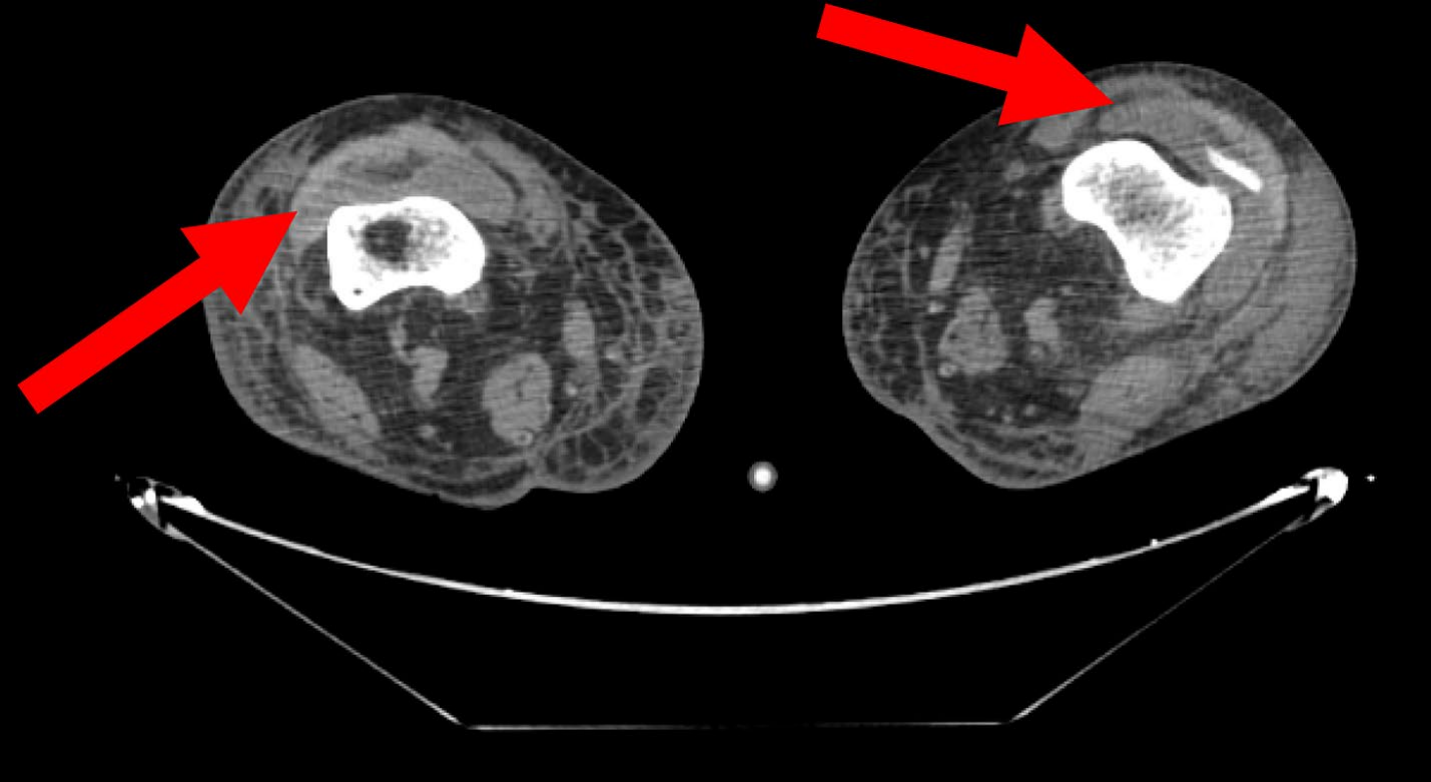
Computed tomography scan of the legs. Horizontal CT scan of the legs, showing effusions at both knee joints (*arrows*).
